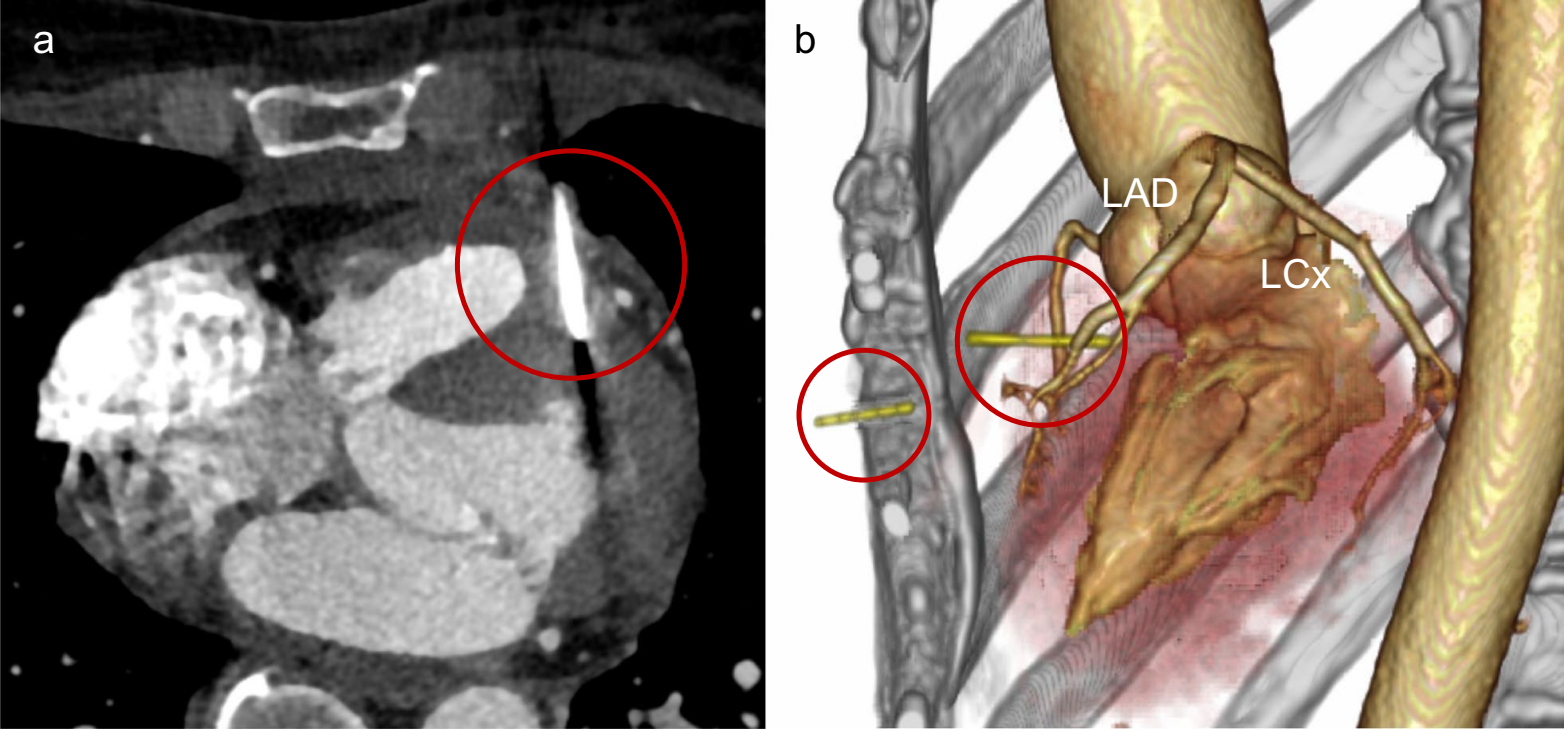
Preoperative CT images. (a) Axial CT showing needle fragment embedded in the LV. (red circle). (b) 3D-CT showing two needle fragments (red circles) sparing both the left internal thoracic and anterior descending artery. LAD, left anterior descending artery; LCx, left circumflex artery
